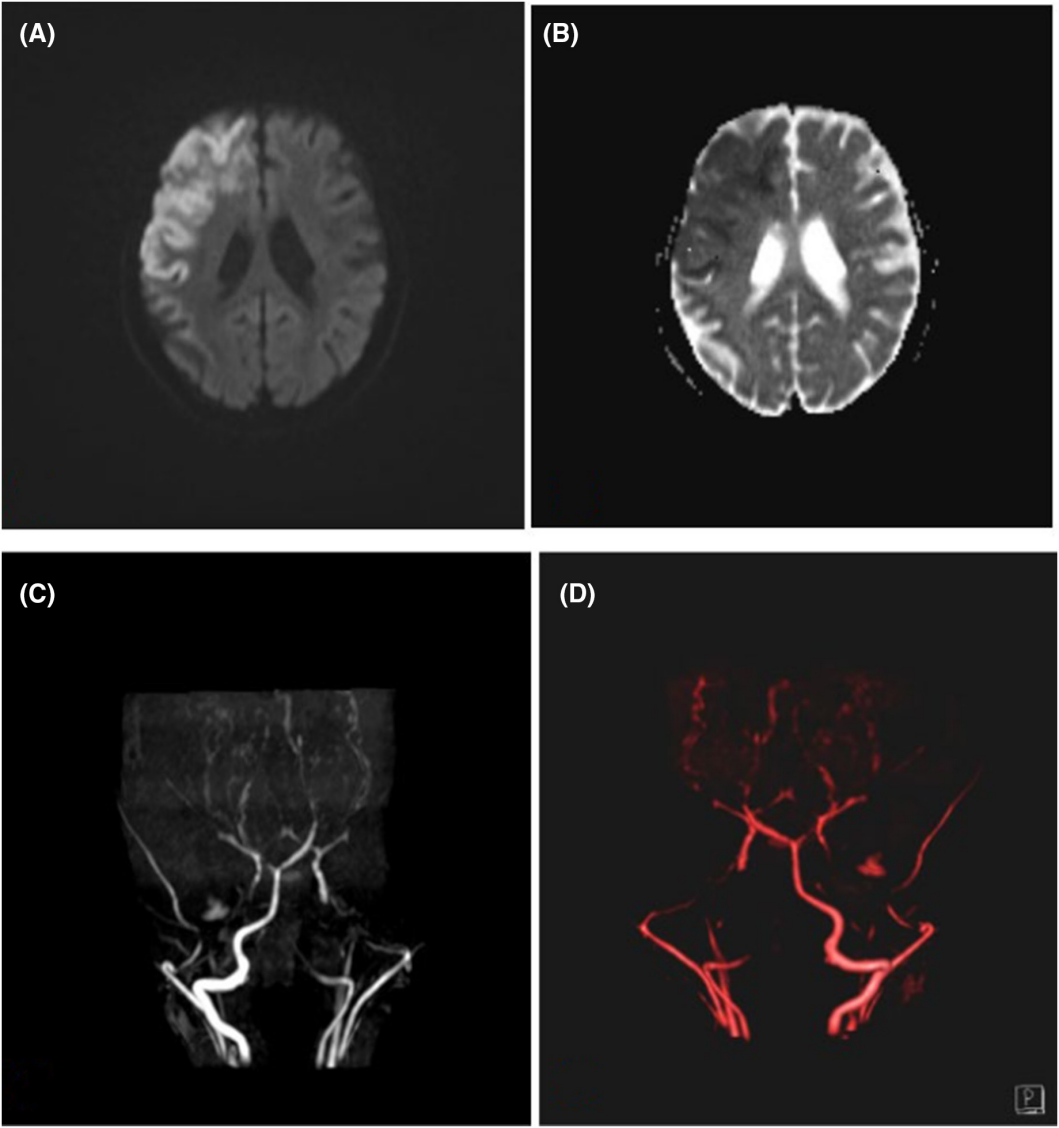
(A, B) Axial MRI images showed a bright area on diffusion weighted images (DWI) with low signals on apparent diffusion coefficient (ADC) maps in favor of acute infarction in territory of right middle cerebral artery. (C, D) Reconstruction images of brain MRA showed extreme narrowing of bilateral long segment of intracranial internal carotid arteries (ICAs), obliteration of the right lacerum segment and bilateral horizontal segment of ICAs with formation of distal non‐moyamoya collateral vessels.
